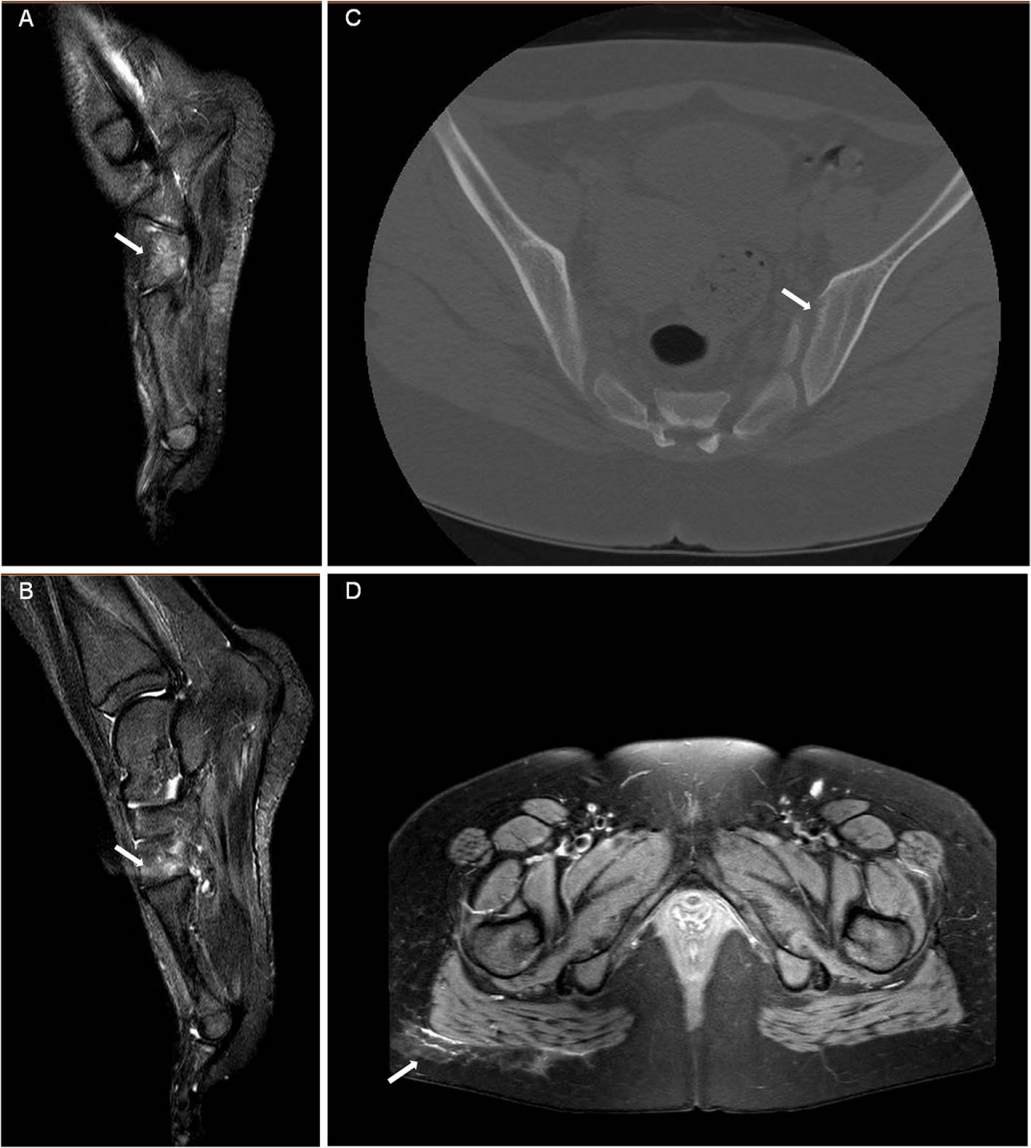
Imagines of left foot, Sacroiliac joint and hip. MRI of left foot showed middle foot involvement with bone marrow oedema in cuboid (A) and cuneiform (B); SIJ CT revealed sacroiliitis with bone destruction (C); MRI of hip showed inflammation in fat layer at the same time (D)
